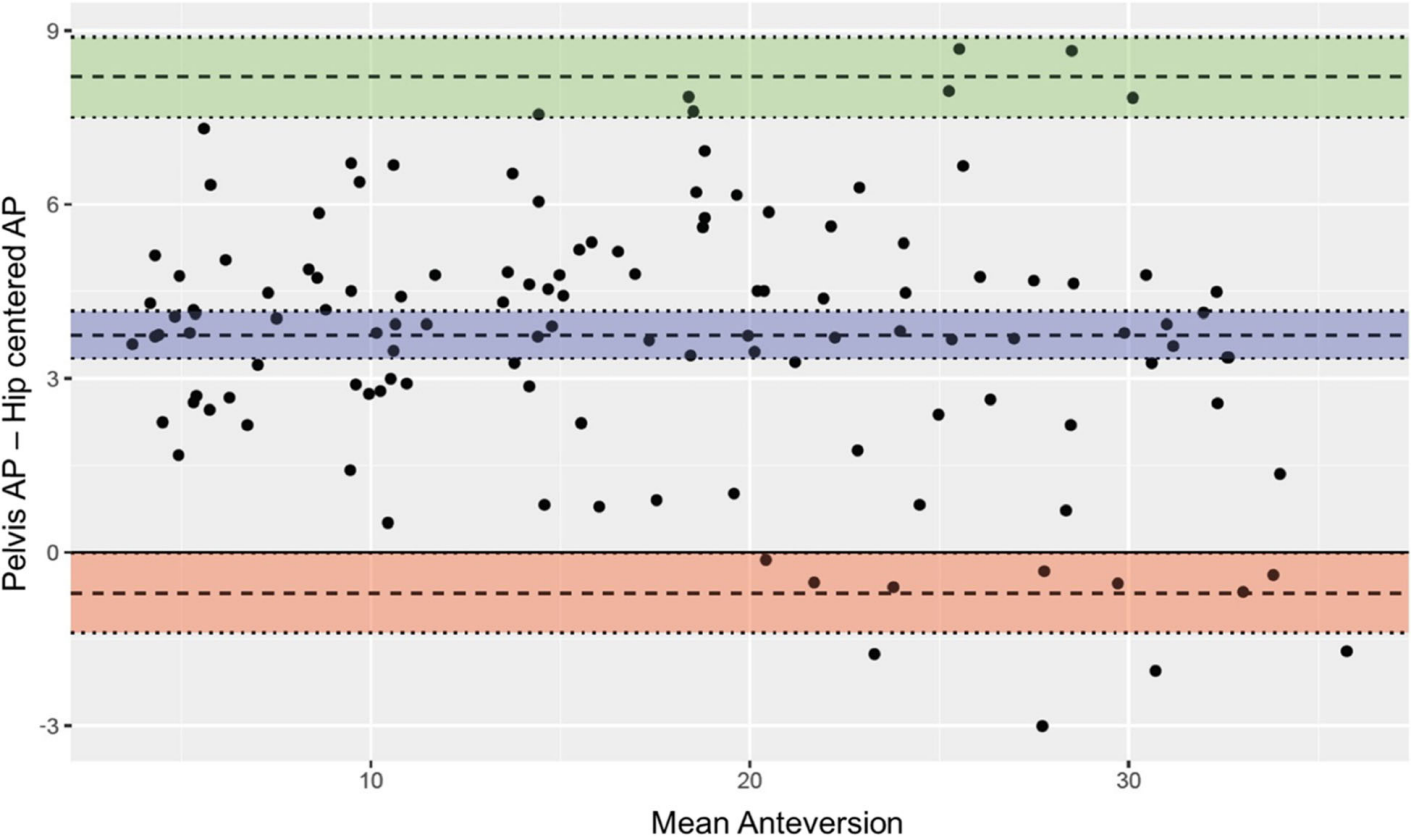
Bland–Altman plots showing differences between anteversion measurements using pelvis AP and hip-centered AP radiographs
